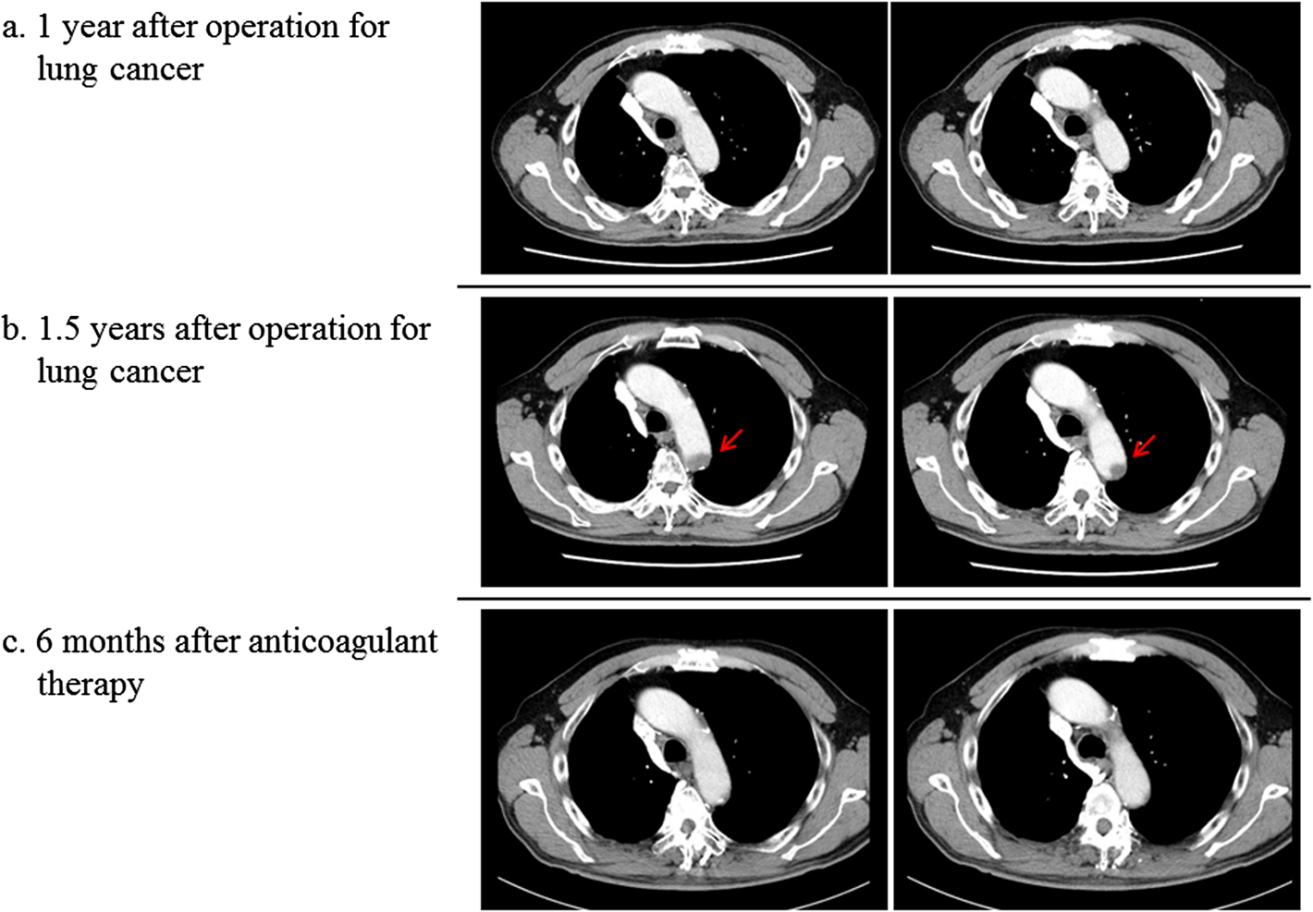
Contrast-enhanced computed tomography (CT) of the chest undertaken 1 years after surgery for lung cancer (a) Contrast-enhanced CT of the chest 1.5 years after surgery for lung cancer. An aortic thrombus was incidentally detected in the distal aortic arch (b) Contrast-enhanced CT of the chest 2 years after surgery for lung cancer. The thrombus in the distal aortic arch has disappeared after 6 months of anticoagulant therapy without an event of symptomatic distal embolism (c) *Arrows* indicate thrombosis
